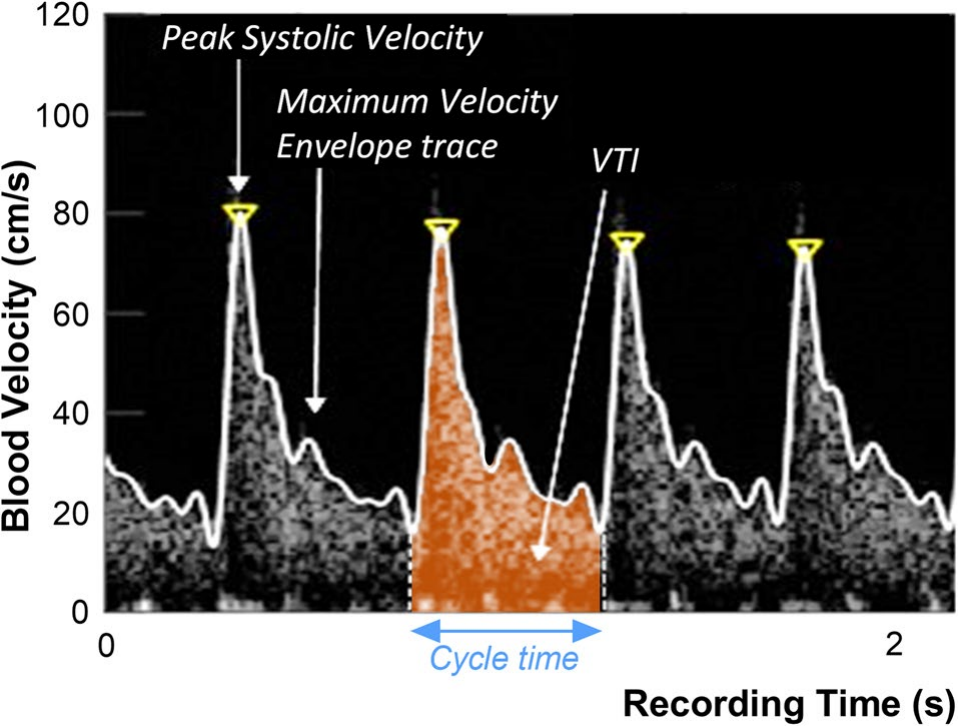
Metrics derived from the spectral Doppler signal for a single cardiac cycle. The velocity time integral (VTI) is the area under the maximum velocity envelope traced from the Doppler spectral waveform for a single cardiac cycle (highlighted in orange) bounded by the cycle time. VTI is also known as the stroke distance and is measured in centimeters.
